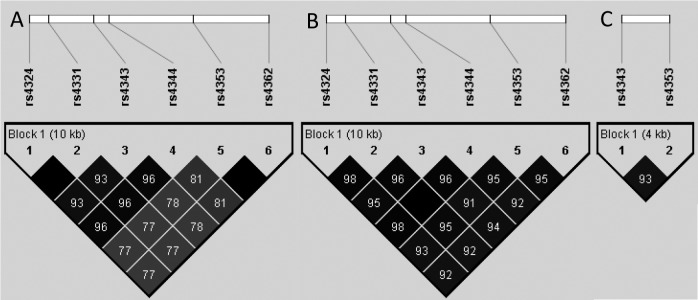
Linkage disequilibrium (LD) relationships between single nucleotide polymorphisms (SNPs) in the genes encoding the angiotensin-converting enzyme (ACE). The top of the figure shows the SNPs. A: HapMap Han Chinese population [15]; B: HapMap European population [15]; C: Echocardiography and Heart Outcome study (ECHOS) population. The strength of LD was determined by R^2^ statistics. The decrease in LD in the Han Chinese population probably reflects the previously reported recombinant breakpoint between rs4344 and rs4353 [12]. No such decrease in LD is observed for the HapMap European population and was also not observed in the ECHOS population. The *ACE* I/D polymorphism was not included in the figure as it has not been genotyped in the HapMap populations [15]. However, this polymorphism is located between rs4331 and rs4343, and is in high LD with these two SNPs [12].
